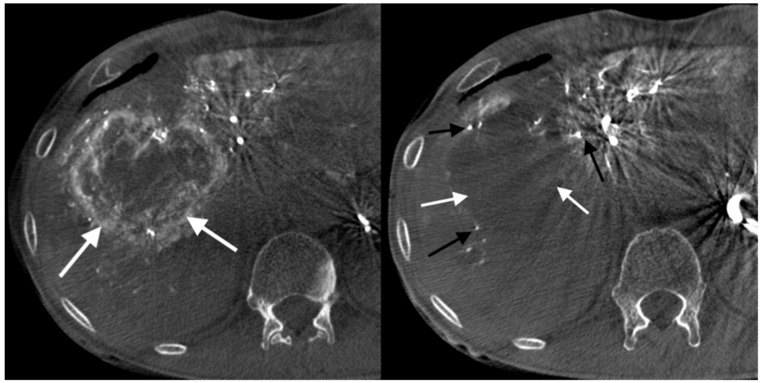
Cone-beam computed tomography image was performed through the SIM catheter in the coeliac artery using 45 mL Iodixanolum (320 mg I/mL) with flow rate of 5 mL/s. Devascularization of a colorectal cancer metastatic liver focal lesion. Left, before the procedure: visible pathological tumor vessels (white arrows). Right, three weeks after the procedure: preserved patency of subsegmental branches (black arrows) and almost complete devascularization of tumor vessels (white arrows).
